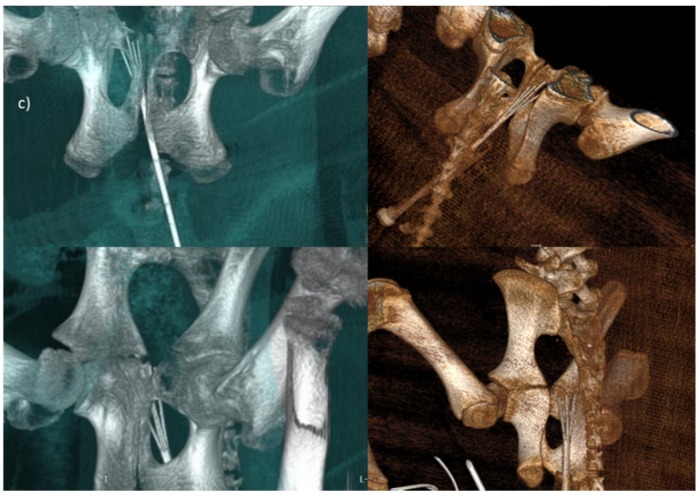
(a) Application on rectum with the trans-anal endoscopic procedure and the 5-needle expandable electrode (20° of divergence; (b) CT transversal sections in the two deployments: on the left, peripheral needles with exposure of 3 cm; on the right side, peripheral needles exposure of 4 cm; (c) volume rendering: in the top, deployment of central needle of 4 cm and peripheral needle exposure of 3 cm; down, central needle and peripheral needles exposure of 4 cm.
